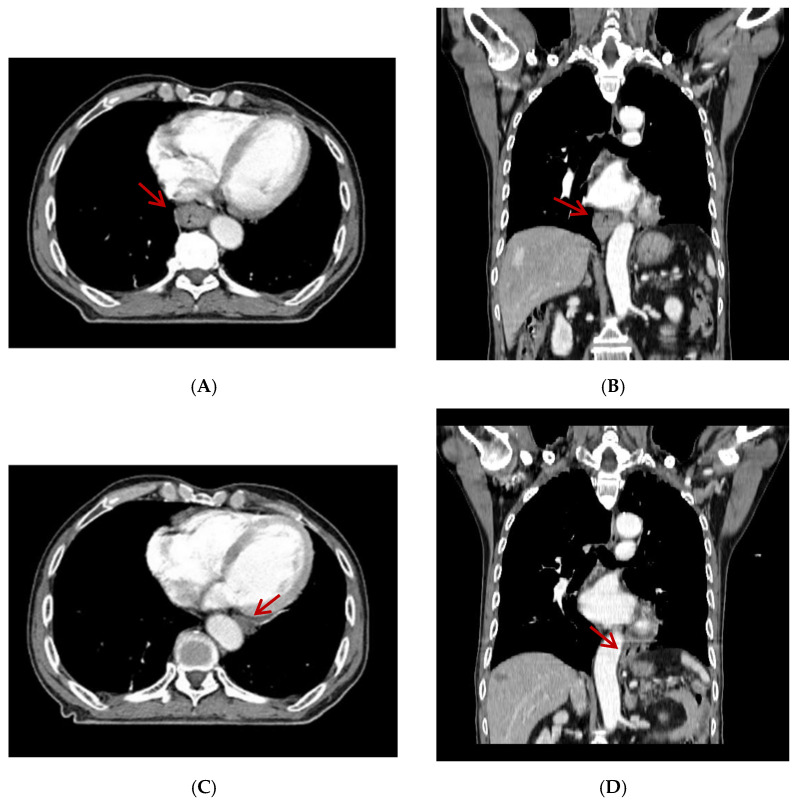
Distal esophageal wall thickening (arrow) was found on axial view (A) and coronal view (B) of initial computed tomography (CT) scan. Metastatic lymph nodes were not seen on these CT slices. Follow-up computed tomography revealed that the esophagus had moved from the right of the aorta to the left on axial view (C) and coronal view (D) (arrow).
